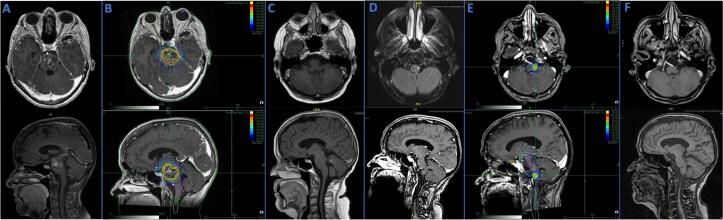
Case example with MRI findings and treatment plans. This case example is about a female patient with HER2 positiv breast cancer initially diagnosed in 2014. In Dezember 2016 this patient experiened paralysis and dumbness on the right body side. A Axial and sagital view, T1 MPRAGE weighted MRI (05/2017). Brainstem metastasis located in the pons with cental necrosis and perifocal edema, 2.6 x 2.0 cm. B Treatment plan for fSRT at the CyberKnife with 30 Gy in 6 fractions at 70 % isodose performed with the CyberKnife, PTV 15.27 ml (05/2017). C Axial and sagital view, T1-weighted MRI (10/2017. The brainstem metastasis showed already a good treatment response 5 months after fSRT. D Axial view,T2-Flair and sagital view T1-MPRAGE weighted MRI (08/2021). After four years new brainstem metastasis located in the medulla oblongata left, 0.2 x 0.2 cm. E Treatment plan for fSRT at the CyberKnife with 30 Gy in 6 fractions at 70 % isodose performed with the CyberKnife, PTV 0.21 ml (08/2021). F Axial view T1 + KM and sagital view T1 MPRAGE weighted MRI (11/2021). Treatment response in the brainstem after three months.
